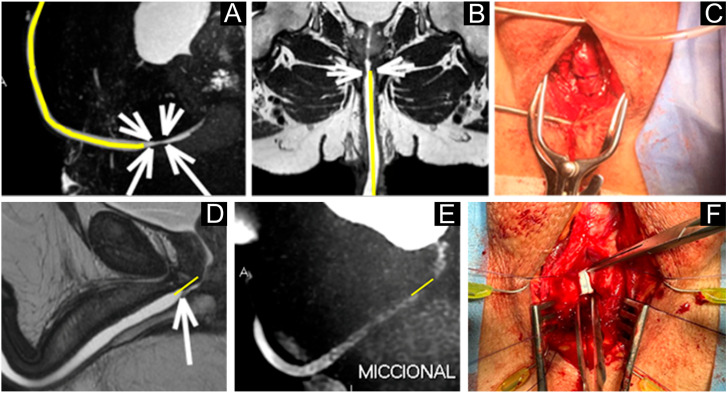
Dynamic magnetic resonance imaging of the urethra in different planes (A–B, D–E) showing the stenotic segment (arrows) and measurements of its length (yellow lines) and caliber. Intraoperative surgical views (C, F) confirm the location and extent of the stenosis, with findings consistent with the measurements obtained by magnetic resonance imaging.
